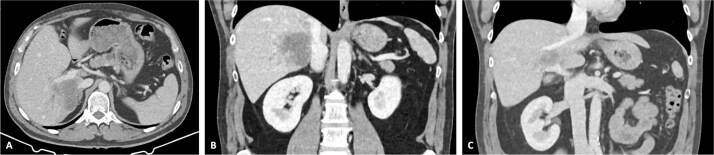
CT scan of the intrahepatic cholangiocarcinoma after chemotherapy. (A) Axial CT scan showing cholangiocarcinoma diameter reduction after chemotherapy and (B) and (C) coronal CT scan view of the vascular relation between the cholangiocarcinoma, IVC, and PV.
